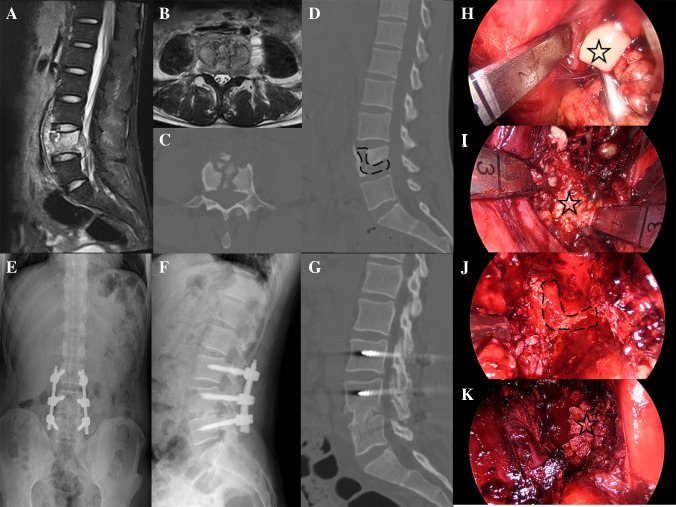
Case 10 A/B: Preoperative MRI revealed abscess both anterior and posterior to the L4, with abscess formation in the left psoas muscle. C/D: Preoperative CT demonstrated severe destruction of the L4, with notable destruction of the posterior vertebral wall .E/F:X-ray at 1 month postoperatively. G:CT at 12 months postoperatively confirmed complete fusion at L4/5. H: Drainage of purulent fluid, with the purulent fluid marked by a black pentagram. I: Exposure of the lesion, with the lesion marked by a black pentagram. J: Preparation of the bone graft bed, with the bone surface indicated by a black dashed line. K: Implantation of allogeneic bone
